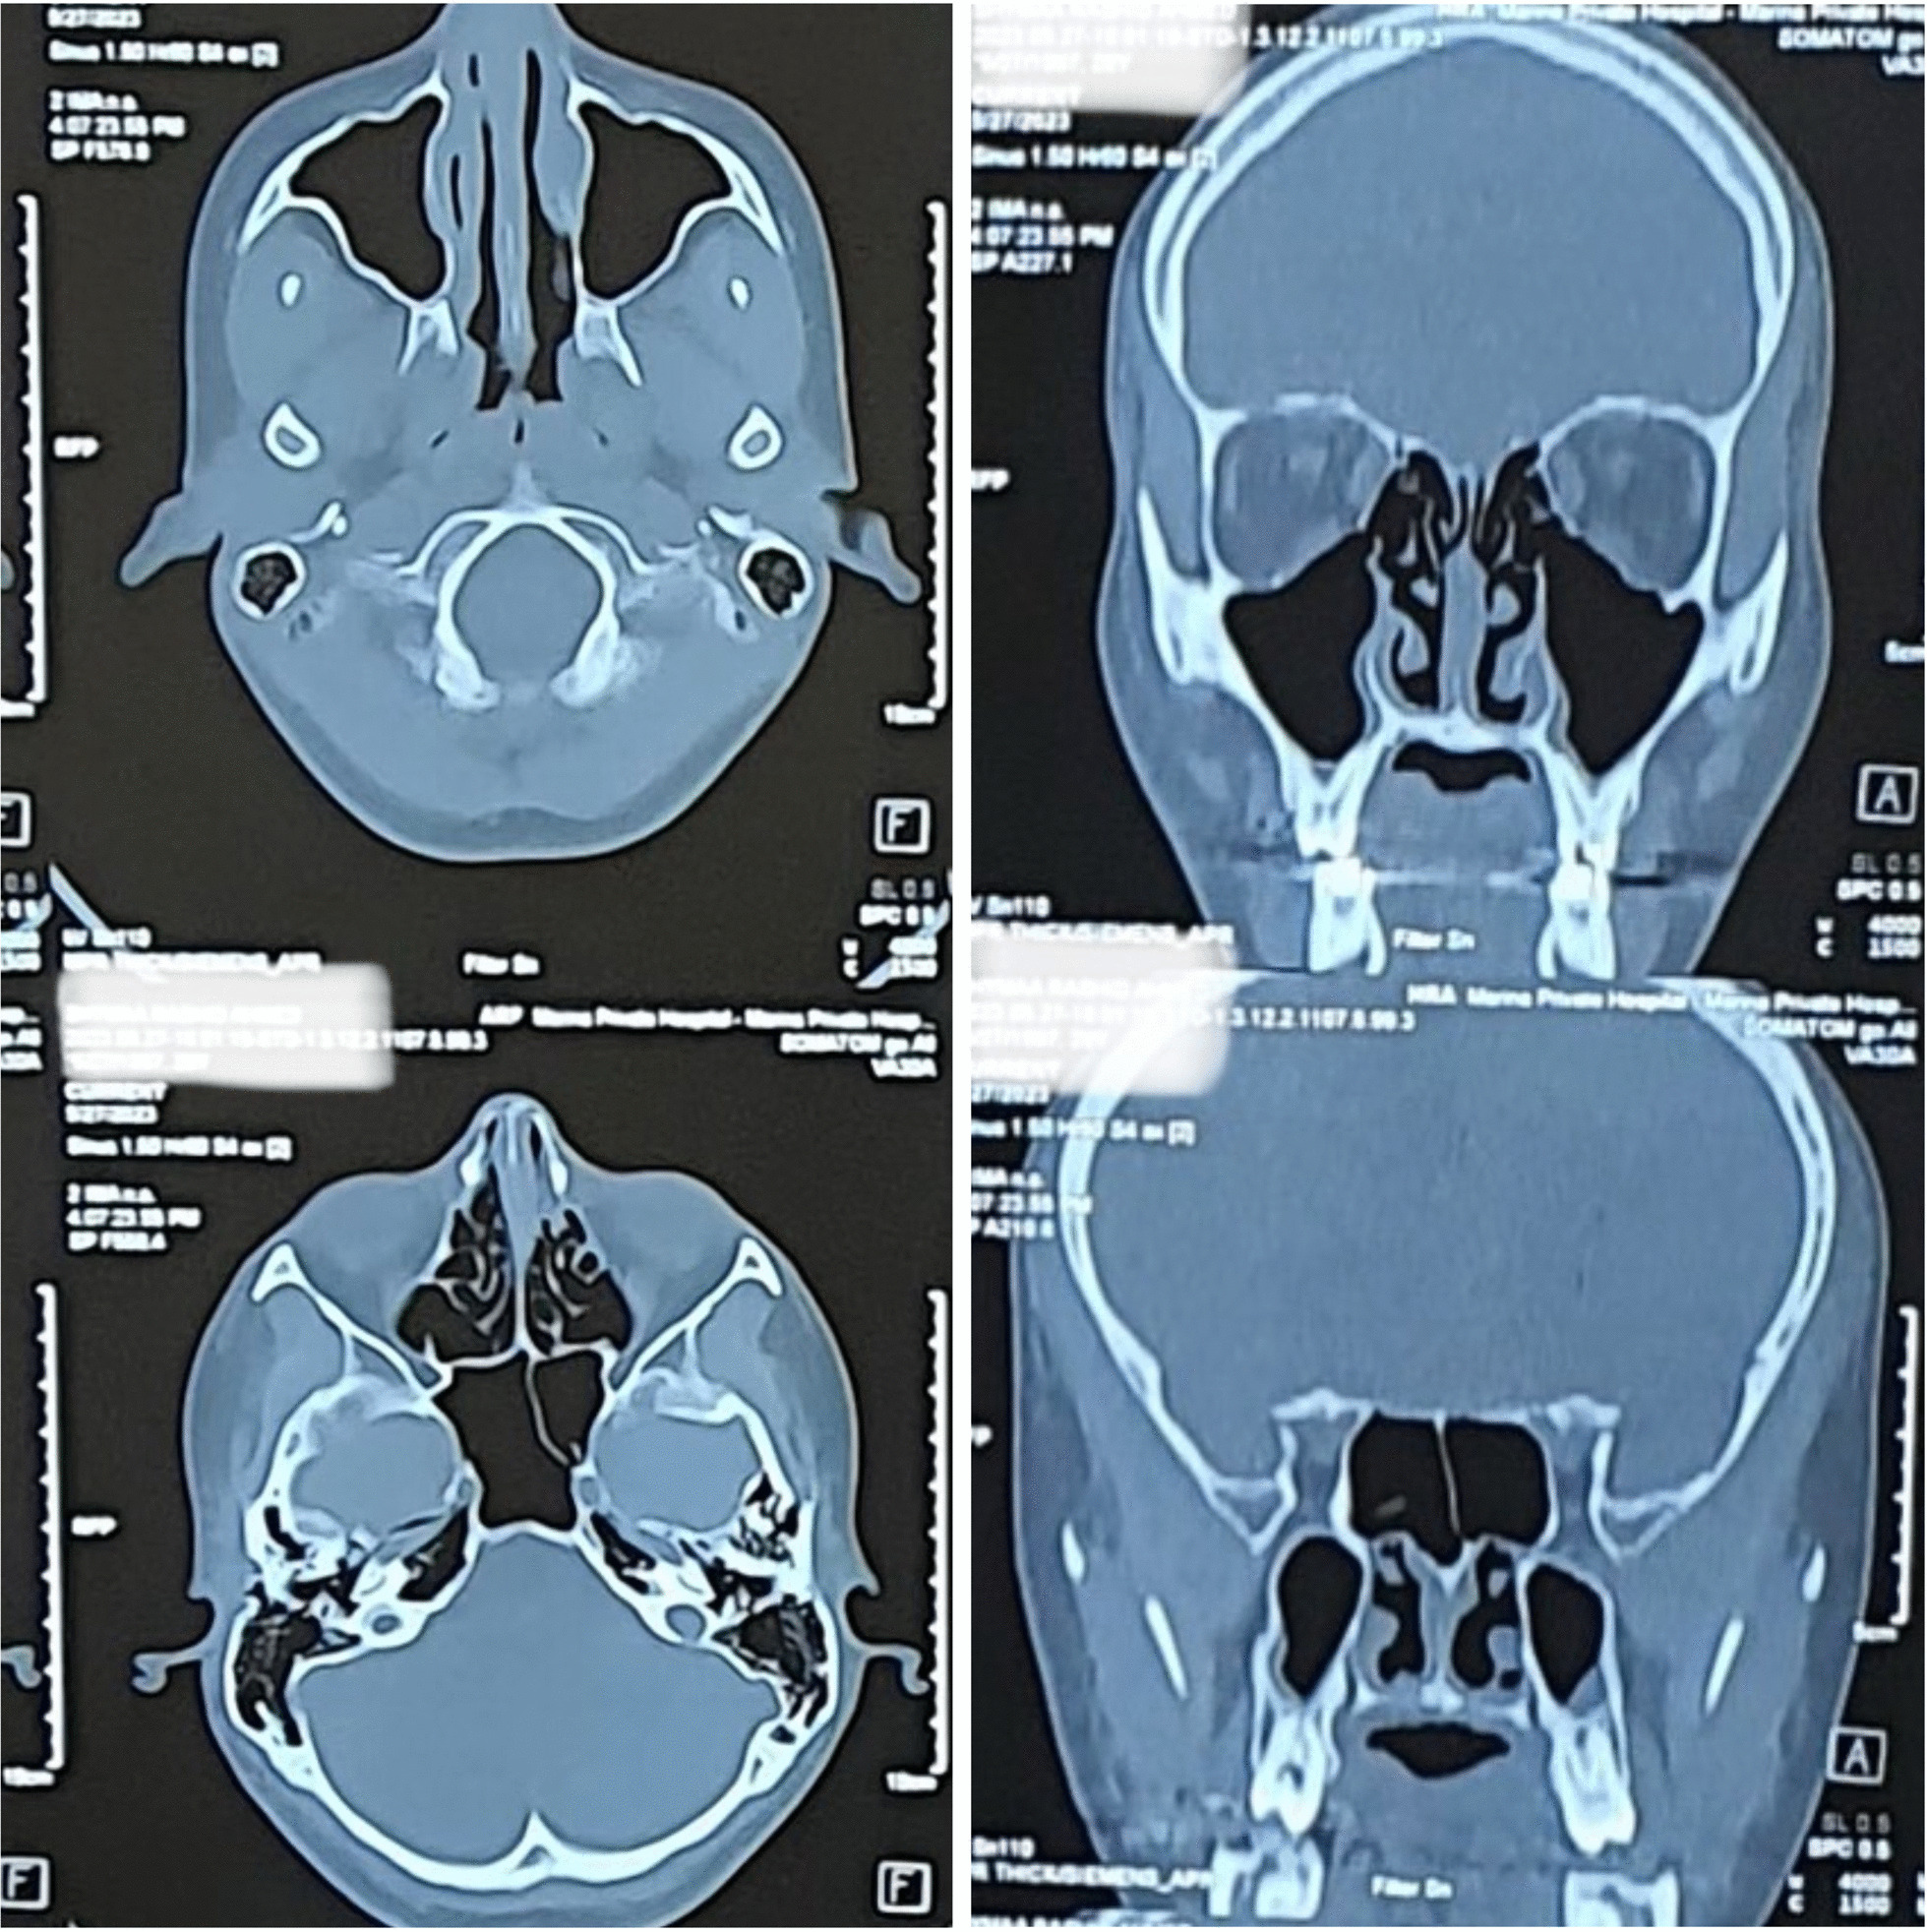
Computed tomography scan of the paranasal sinuses
